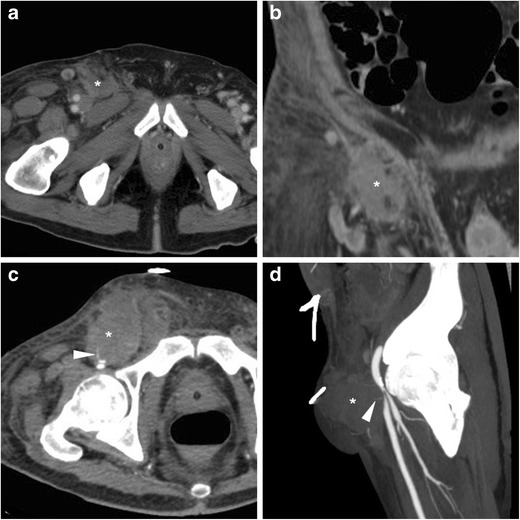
Delayed groin bleeding in a 70-year-old male patient after transarterial mitral and aortic valve replacement. Early CT (A, B) showed circumscribed effusion (*) abutting the CFA at the level of percutaneous access, which was interpreted as a minor bleed and treated conservatively. However, during hospitalisation for infected sternal dehiscence, repeated CT angiography (C, D) showed development of a 4.5-cm groin haematoma (*) containing a thin contrast medium (CM) extravasation focus (arrowheads), causing compression on the CFA (MIP image D) which required stenting
